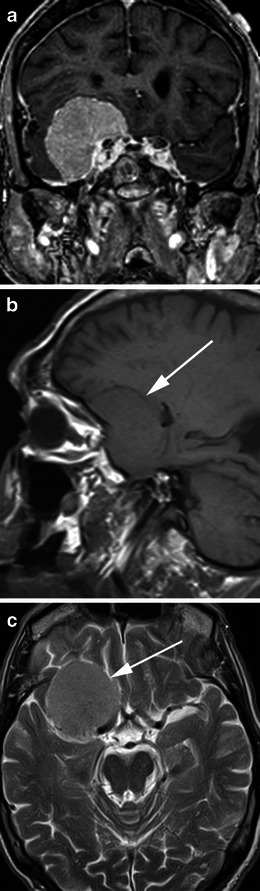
a Post-contrast coronal T1-weighted sequence in an 80-year-old woman with a 1-month history of confusion demonstrates a well-circumscribed, homogenously enhancing mass within the floor right middle cranial fossa with a broad dural tail that inferomedially contacts the right cavernous sinus. The right temporal lobe is compressed superiorly by the mass without invasion. The lesion was proven at surgery to be a meningioma. b Sagittal T1-weighted sequence in the same patient as a demonstrates the mass (*arrow*) to be isointense to grey matter and to extend anteriorly along the posterior aspect of the anterior cranial fossa floor. c Axial T2-weighted sequence in the same patient as a demonstrates the mass (*arrow*) to be mildly, homogeneously hyperintense to grey matter
